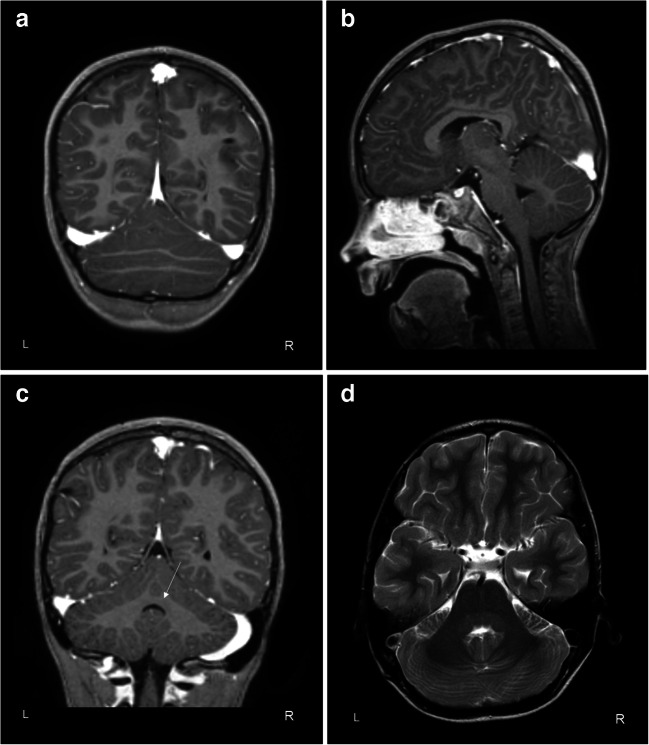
Eight-year-old male patient with rhombencephalosynapsis. Coronal (a) and sagittal (b) images of the T1-weighted MRI scan show the fused cerebellar hemispheres and absent vermal midline structure. The nodulus (lobule X) is shown on sagittal slice of the T1-weighted MRI scan (c) and an axial slice of the T2-weighted MRI scan depicting the deep cerebellar white matter (d)
